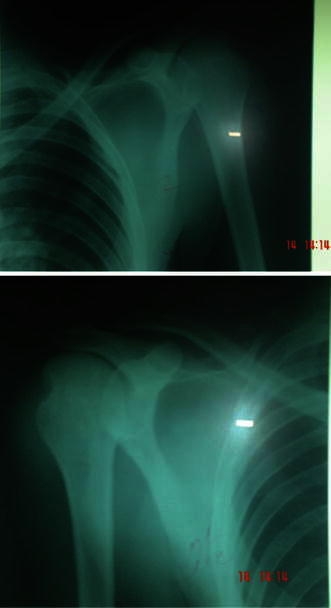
Check X-rays showing reduced joints
